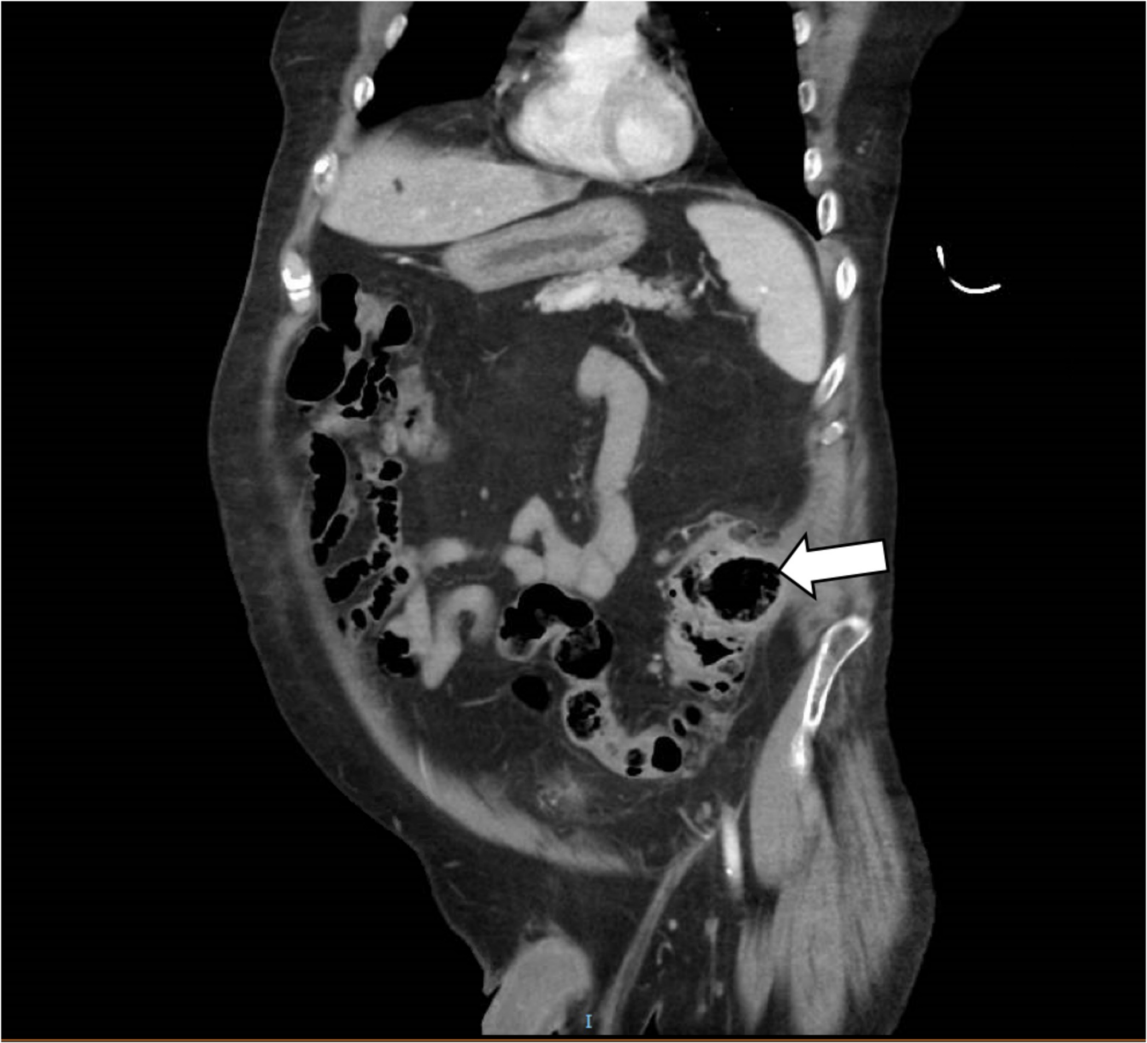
Coronal view of an enhanced abdominal CT scan showing a large air-filled thin-walled cavity arising from the sigmoid colon (arrow)
